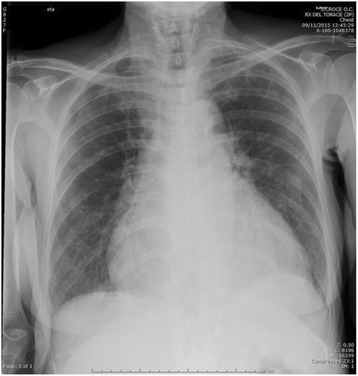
Chest x-ray of the same patient showing enlarged cardiac shadow and uncertain signs of pleural effusion and interstitial lung disease
